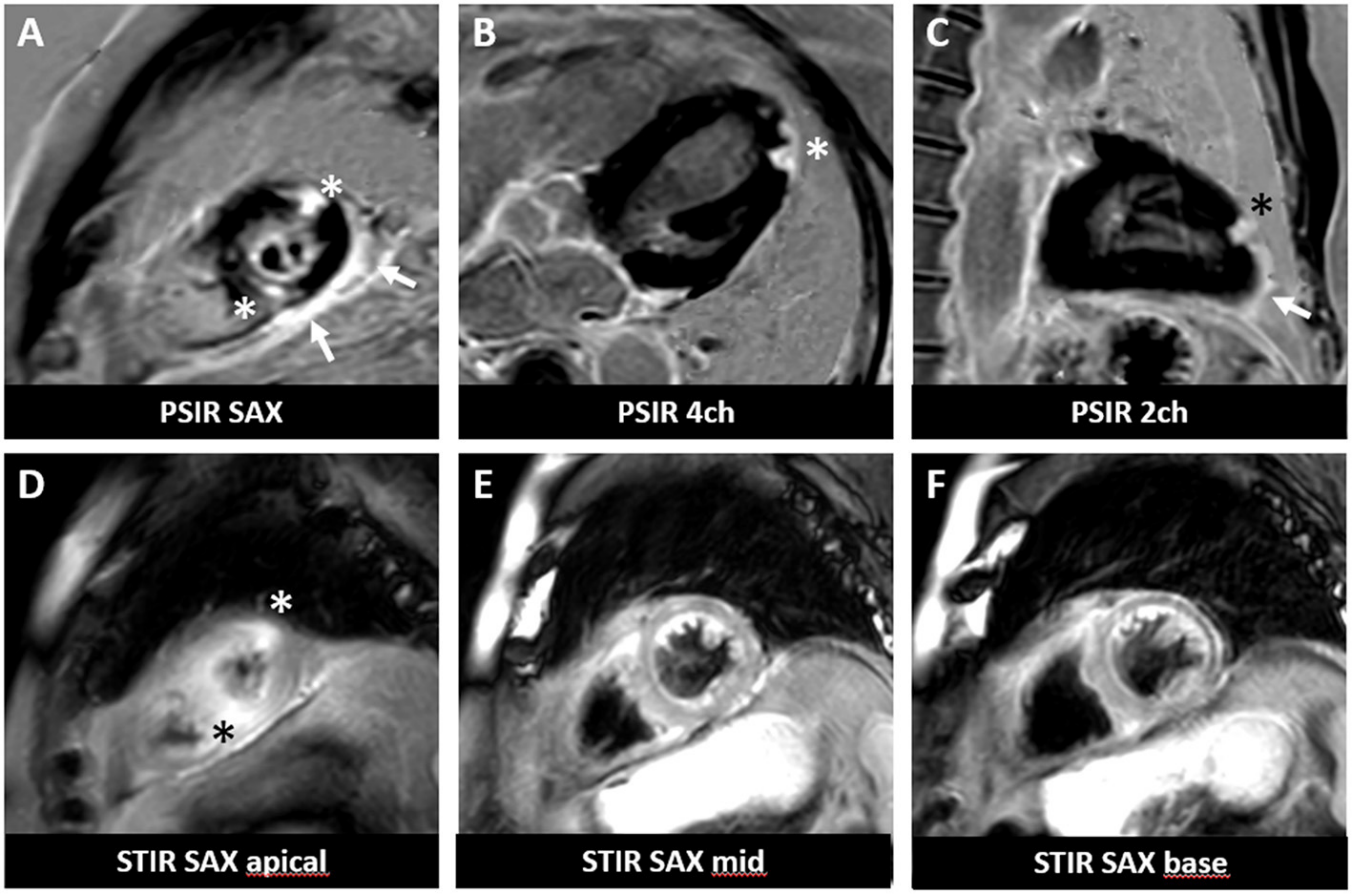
Cardiac magnetic resonance imaging of a patient with irM following a single infusion of cemiplimab. Cardiac MR revealed focal subepicardial to mid myocardial delayed gadolinium enhancement (A–C) associated with edema (D–F) at the lateral and inferoseptal apex (asterisks) involving the pericardium (arrows) in a delayed gadolinium enhancement sequence performed according to clinical standard. PSIR, phase-sensitive inversion recovery; STIR, short tau inversion recovery; SAX, short-axis view; 4ch, 4-chamber view; 2ch, 2-chamber view.
